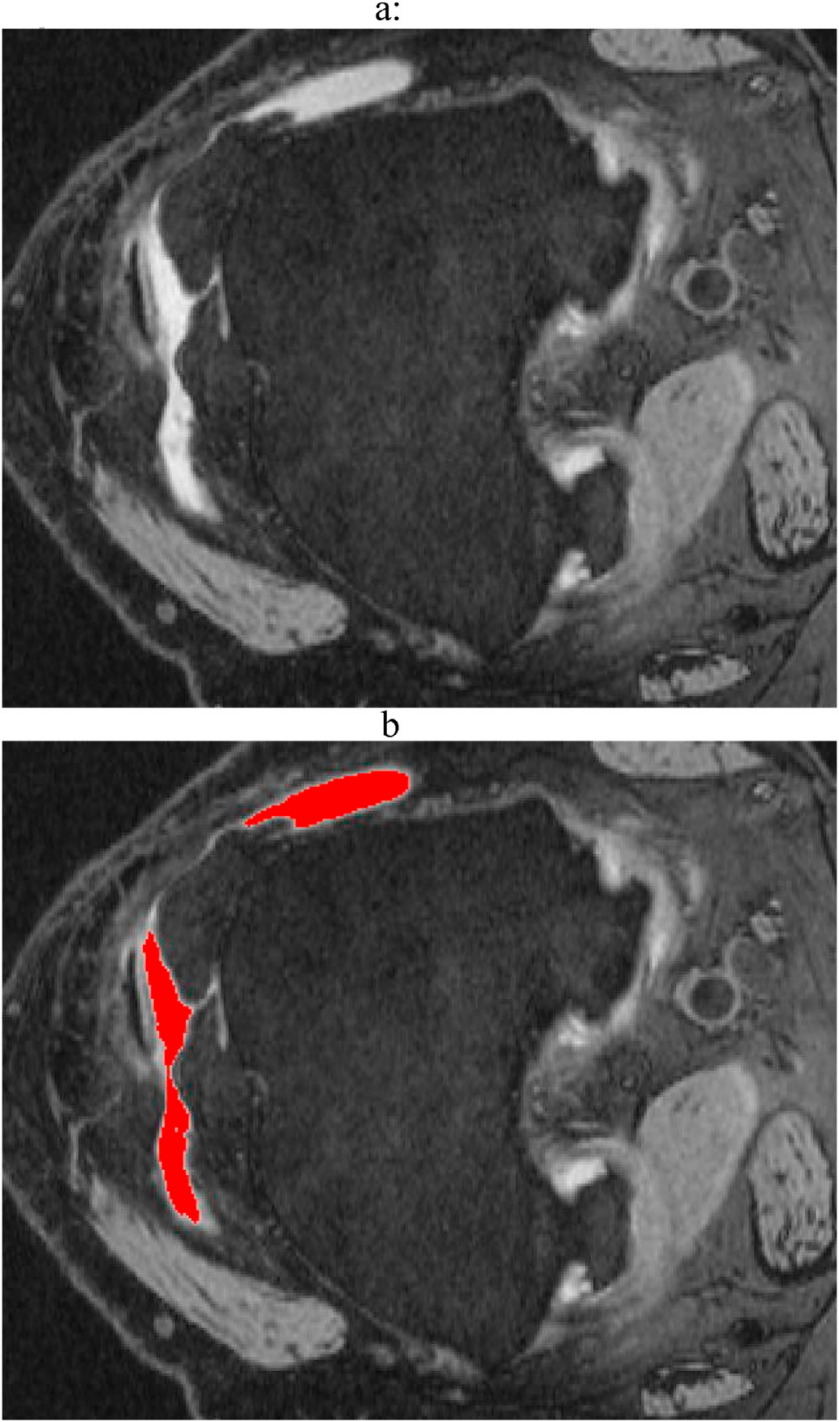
Example of the software joint effusion-synovitis (ES) measurement on MRI (a) Axial MR image of the knee at the level of the suprapatellar bursa depicting bright increased signal intensity fluid bursa fluid and edema. (b) The computer uses a thresholding method to identify the regions of joint effusion/synovitis which are shaded in red.
